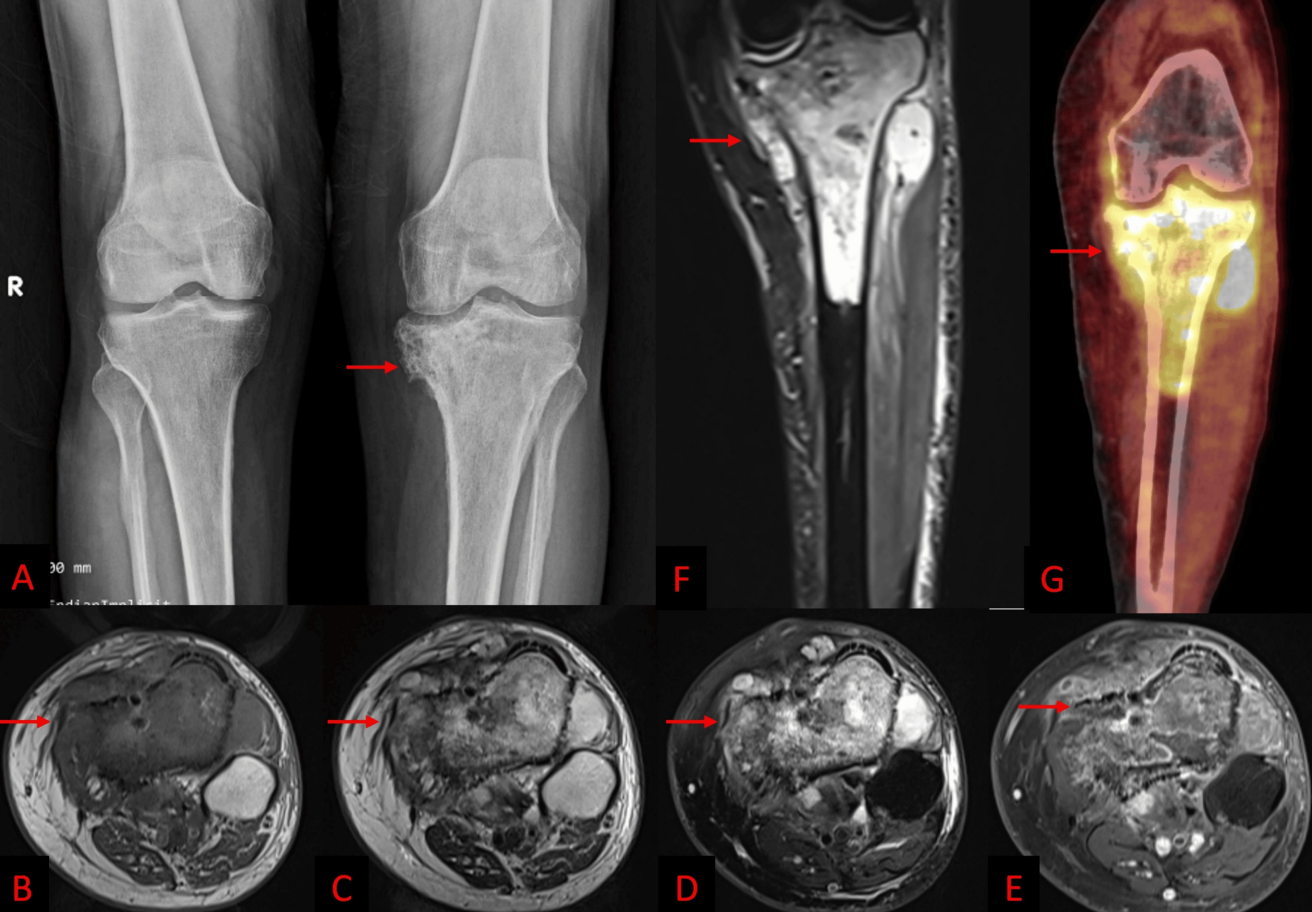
Anteroposterior radiograph of bilateral knees (A) shows a lytic lesion with irregular margins and sclerosis in the medial condyle of the tibia (red arrow). Axial T1-weighted (B), T2-weighted (C), fat-saturated T2-weighted (D), and post-contrast T1-weighted (E) MRI images show a heterogeneous lesion in the proximal tibia with enhancement and extraosseous soft tissue along the medial cortex (red arrows B-E). Coronal fat-saturated T2-weighted MRI (F) shows a well-defined lesion in the epiphyses and metaphysis of the tibia with extraosseous soft tissue (red arrow). Coronal PET-CT image (G) shows a hypermetabolic lesion in a similar region (red arrow). MRI: magnetic resonance imaging, PET-CT: positron emission tomography-computed tomography
